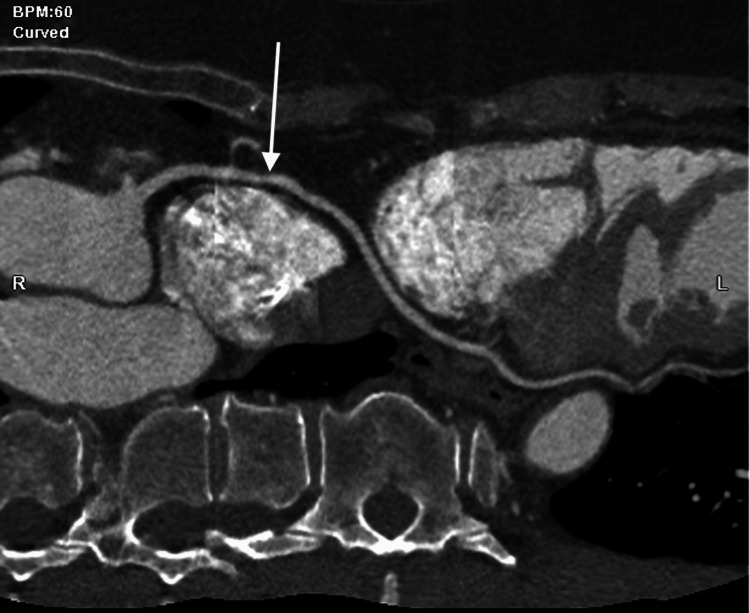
2D curved multiplanar reconstruction Two-dimensional curved multiplanar reconstruction from coronary computed tomography angiography (CTA) demonstrating a large anomalous left main coronary artery (LMCA) (white arrow) arising from the right coronary ostium. The vessel is shown coursing superiorly and anteriorly to the pulmonary artery, consistent with a low-risk anterior trajectory without evidence of an inter-arterial or intramural course.
